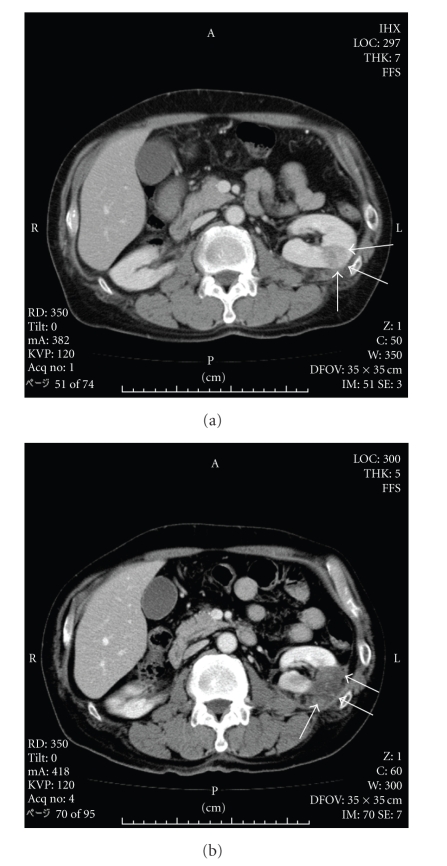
(a) Contrast-enhanced CT showed a solid tumor of 2.5 cm in diameter with weak enhancement in the left kidney (case number 5). (b) The tumor was replaced by low-density tissue without enhancement following RFA, and he was free of disease postoperatively for 12 months.
